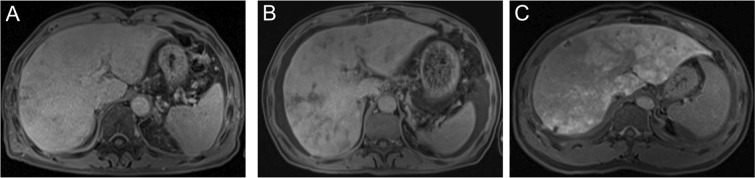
The distribution of hypointensity in hepatobiliary phase of gadoxetic acid-enhanced MRI. (A) Multifocal distribution (mild); (B) multifocal distribution (severe); (C) diffuse distribution.
